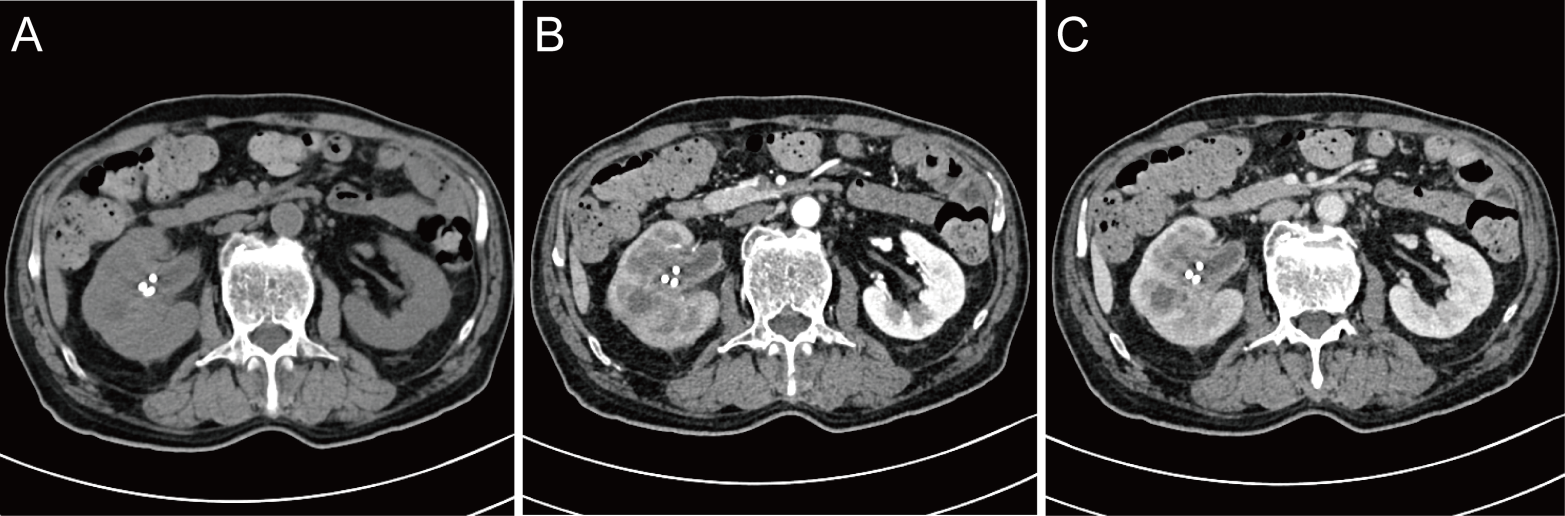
Review of enhanced CT after nephrostomy. (A) plain scan; (B) arterial phase; (C) venous phase.
